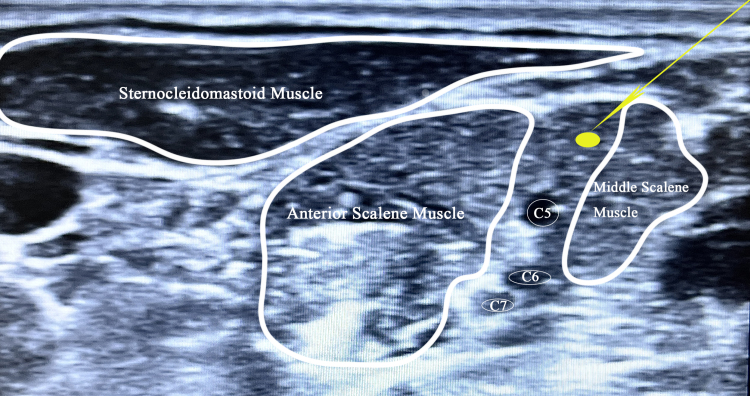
Ultrasound-guided phrenic nerve block puncture. The puncture needle (yellow arrow) is advanced toward the phrenic nerve (yellow dot) under ultrasound guidance. Surrounding anatomical landmarks, including the sternocleidomastoid, anterior scalene, and middle scalene muscles, are shown for reference. The use of this image has been approved by the patient with written informed consent.
